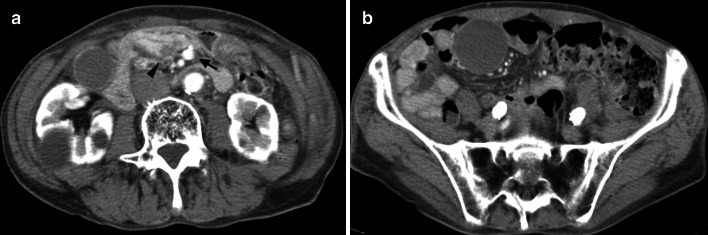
Computed tomography findings. a A 25-mm low-density mass located in the pancreatic head (arrowhead). The superior mesenteric vein (SMV) was located to the left of the superior mesenteric artery, showing the SMV rotation sign (arrow). b The small bowel was located in the right abdomen, and the large bowel was located in the left abdomen
